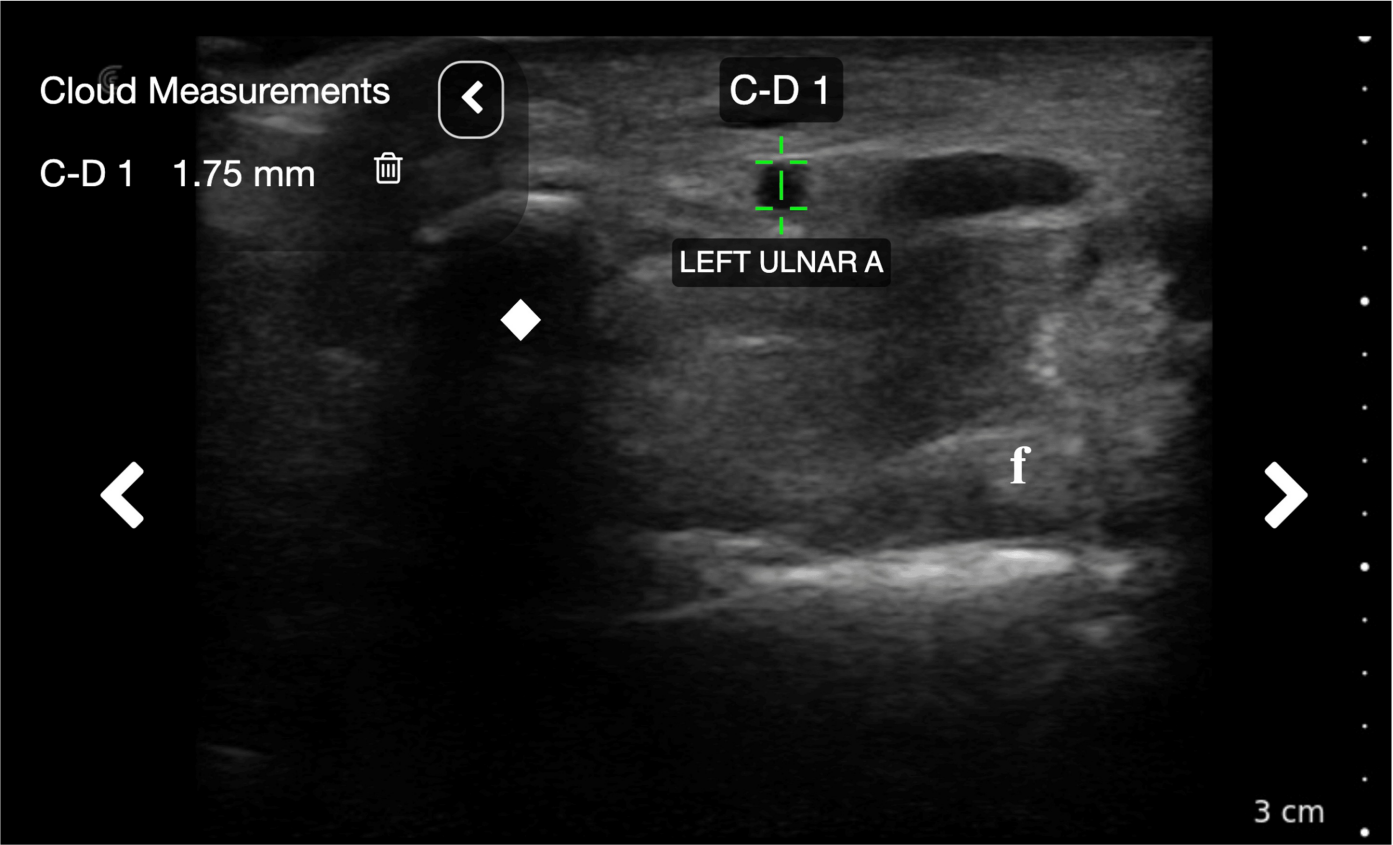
Ultrasonographic arterial measurement Utilization of Clarius virtual caliper function (green crosshairs) to measure internal arterial diameter. The left ulnar artery is demonstrated in this image. diamond: pisiform bone; f: flexor tendon
